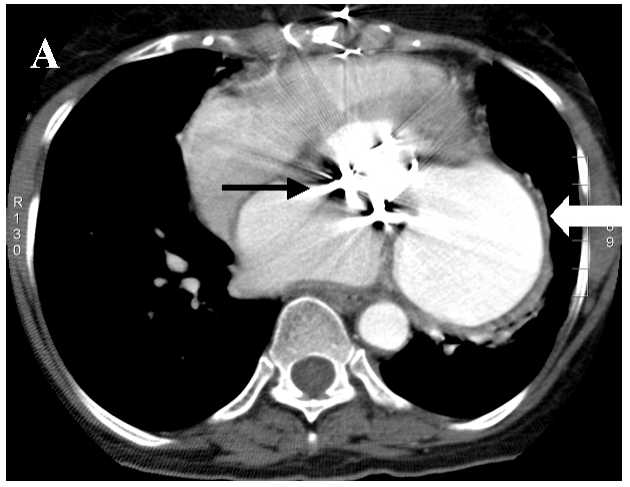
Axial source image of CT angiography showing the annular extension of the tear (thick white arrow -pseudoaneurysm, black arrow- valve).
